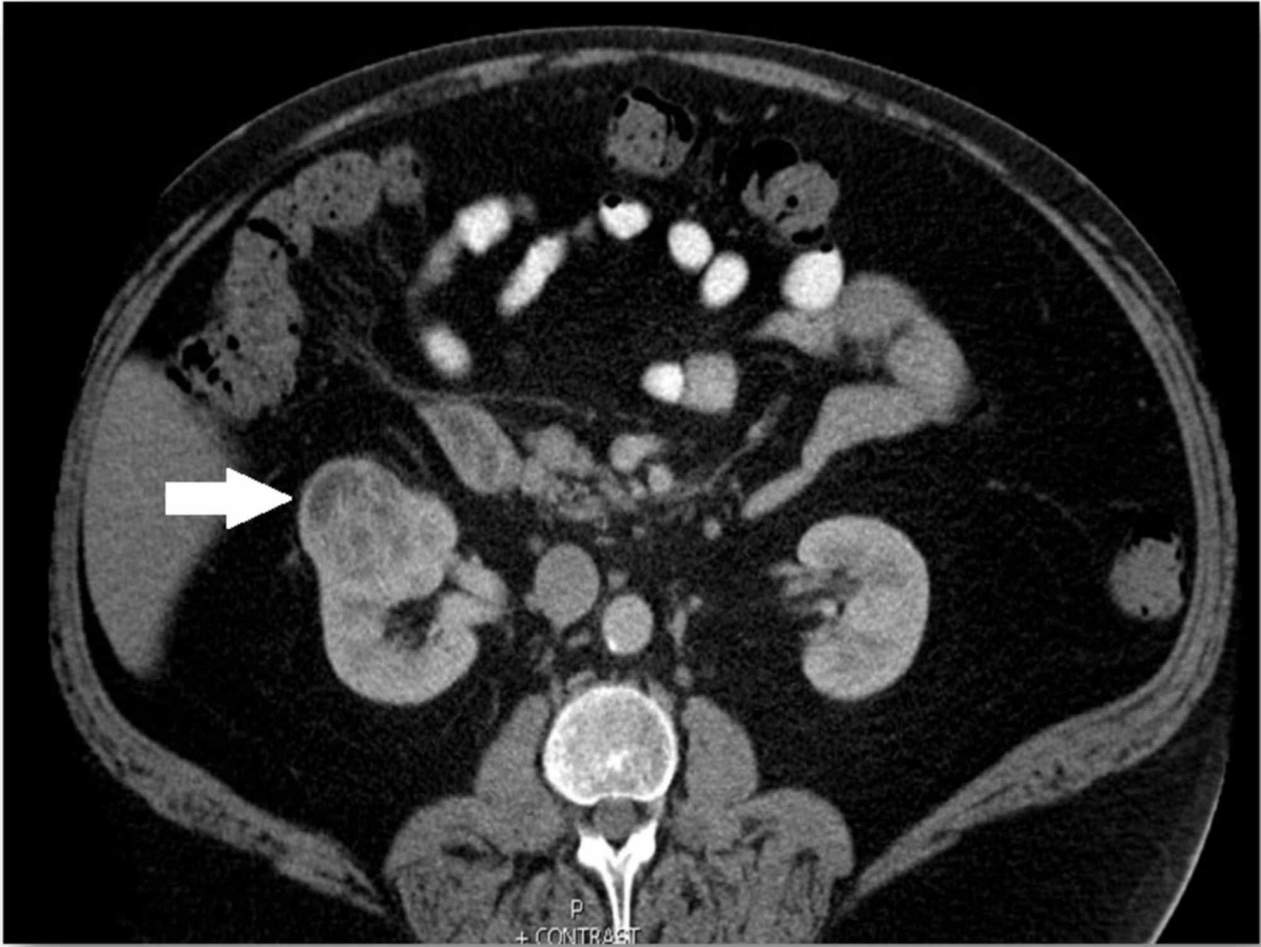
Computed tomography abdomen showing an exophytic mass originating from upper lateral aspect of the right kidney
